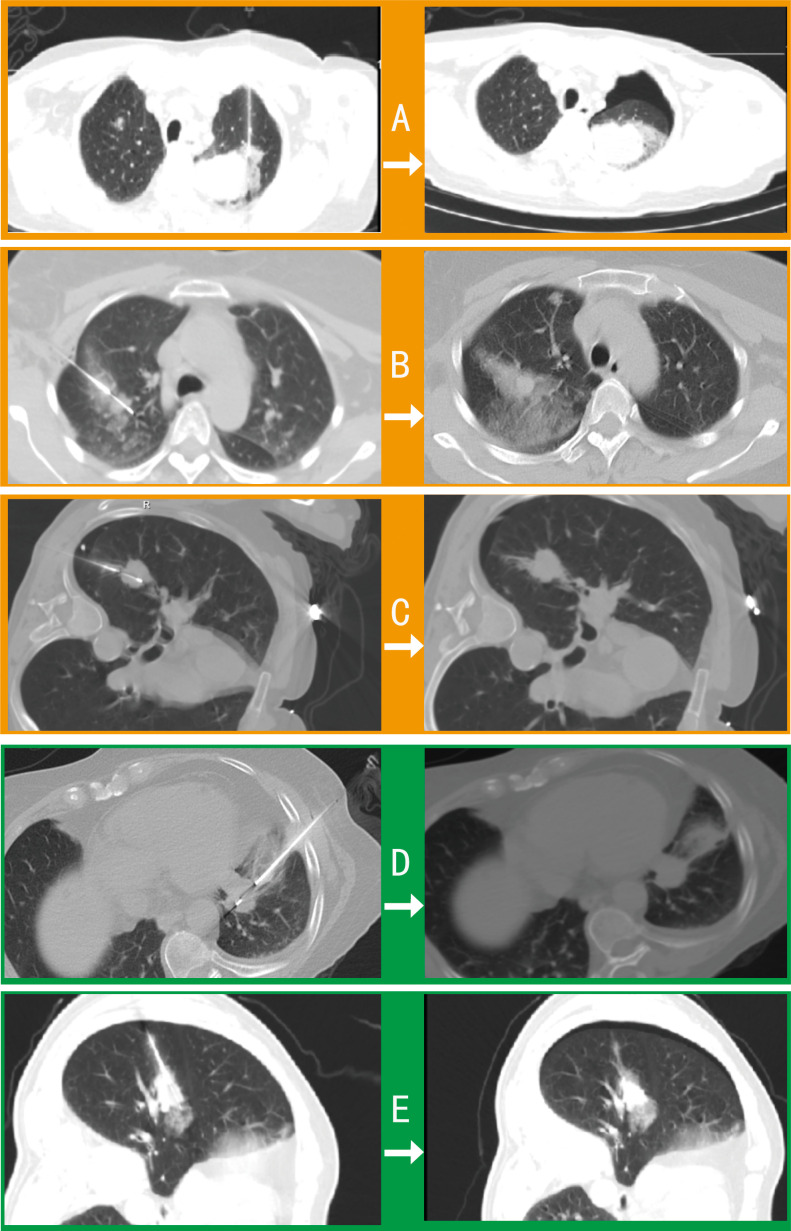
Comparative imaging of post-biopsy complications in pulmonary procedures. Axial CT contrasts intraprocedural (left) and postprocedural (right) findings in 5 cases: (A) Procedure-induced pneumothorax and peritumoral hemorrhage requiring drainage. (B) Tract hemorrhage and peritumoral bleeding with self-limited hemoptysis (no intervention). (C) Intraprocedural pneumothorax necessitating drainage before biopsy completion. (D) Asymptomatic tract hemorrhage. (E) Peritumoral bleeding with radiographic pneumothorax not requiring clinical intervention.
